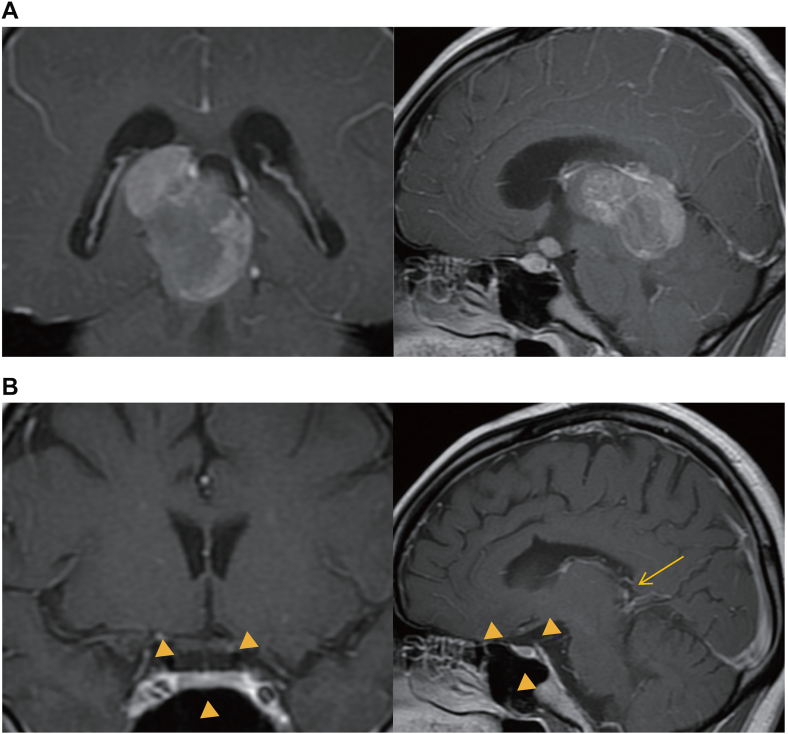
MRI findings at initial diagnosis and before conception. (A) MRI at initial diagnosis showing the germinoma in the pineal and suprasellar regions. (B) Preconception MRI showing resolution of the tumor after treatment. Arrowheads indicate the pituitary stalk and sella, while the arrow indicates the treated pineal lesion; the optic chiasm shows no compressive changes. *MRI* = magnetic resonance imaging.
